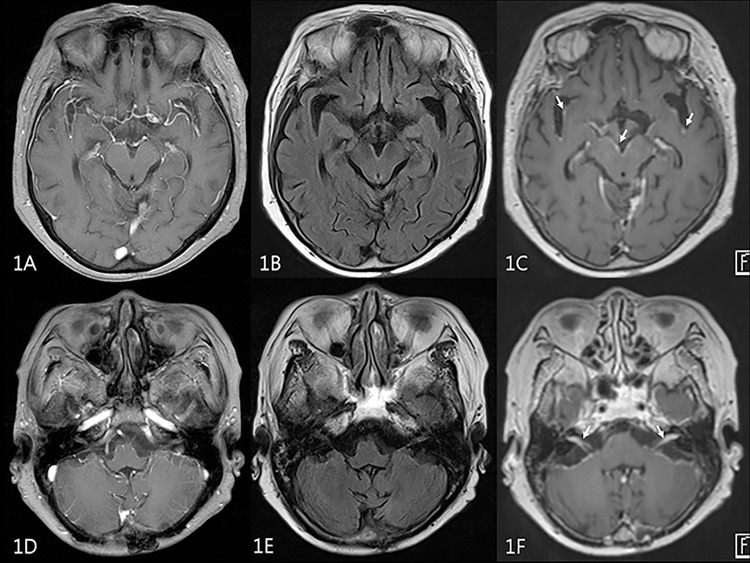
MR images of 72 year-old female patient with breast cancer. 2D contrast-enhanced T1-weighted GRE (A,D) and 2D FLAIR (B,E) were negatively interpreted by a trainee rater. On 3D contrast-enhanced T1-SPACE (C,F), leptomeningeal enhancement along interpeduncular cistern, sylvian fissures (arrows on 1C) and internal auditory canals (arrows on 1F) was seen.
